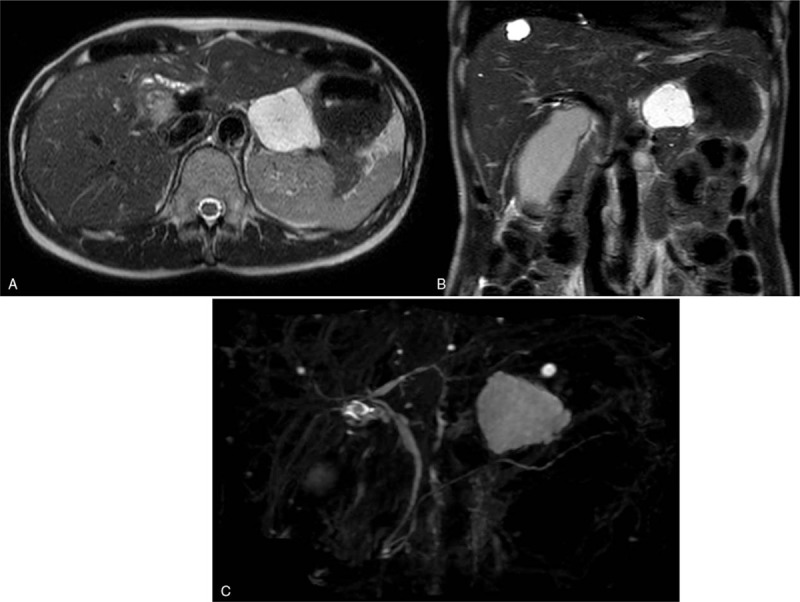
Magnetic resonance imaging findings. Multilobulated cyst with thin internal septum spreading out from pancreatic tail does not appear to communicate with main pancreatic duct. (A) Axial and (B) coronal image and (C) Magnetic resonance cholangiopancreatography.
